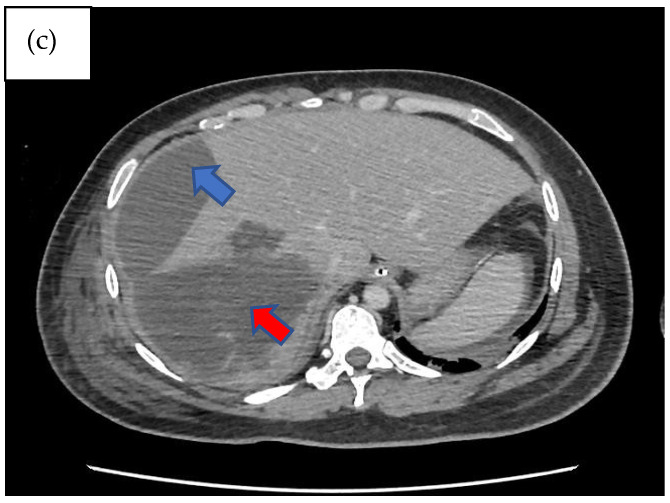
Computed tomography scan of the abdomen non-contrasted phase showing heterogenous areas consisting of hematoma and laceration at segments V, VI, VII, and VIII extending down to the subhepatic region (red arrow). Inferiorly, it extends down to the hepatic flexure, just lateral to the right pararenal space. Another subcapsular collection was seen adjacent to right liver lobe (blue arrow): (a) coronal view, (b) sagittal view, and (c) axial view, with findings as aforementioned.
